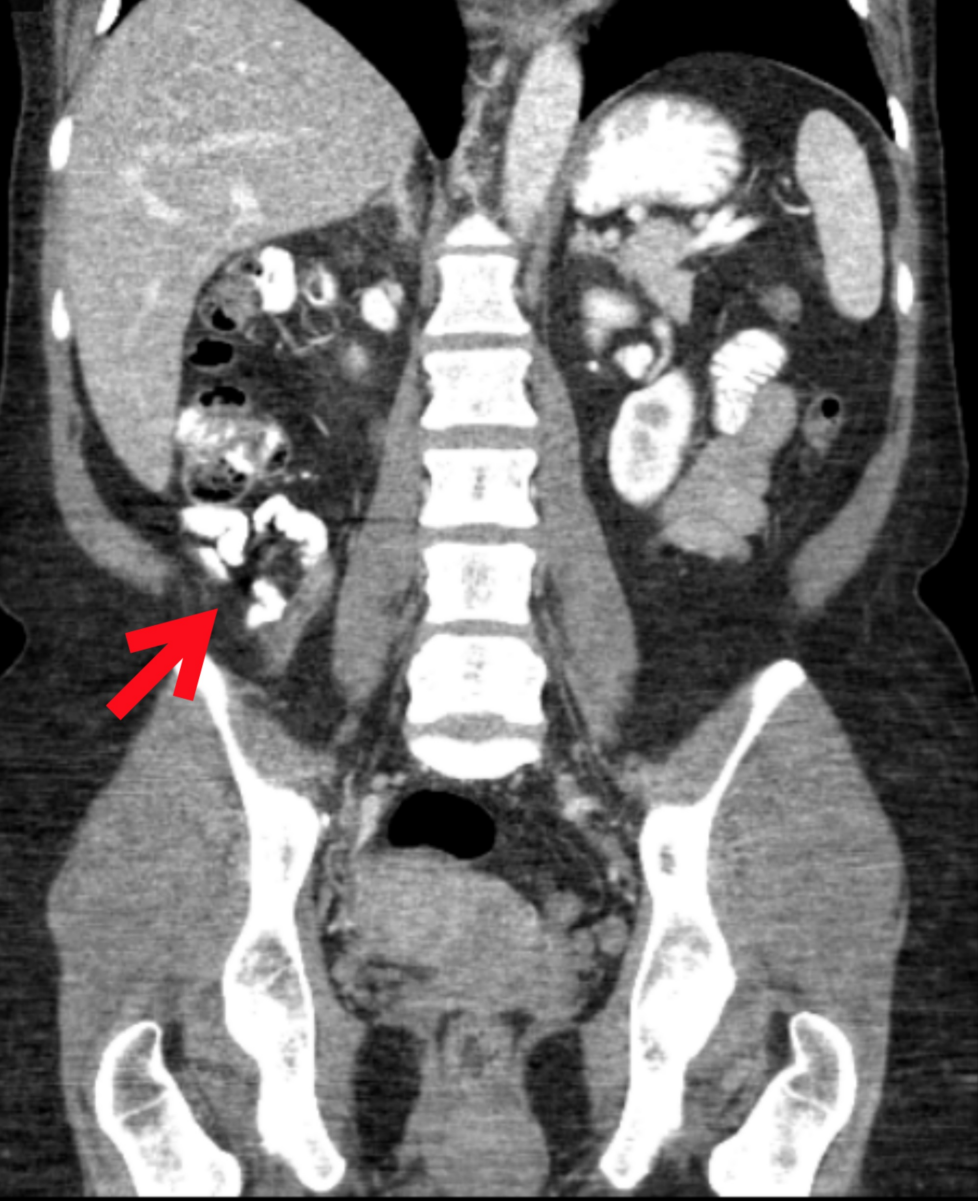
Coronal CT scan demonstrating appendicitis CT: computed tomography
